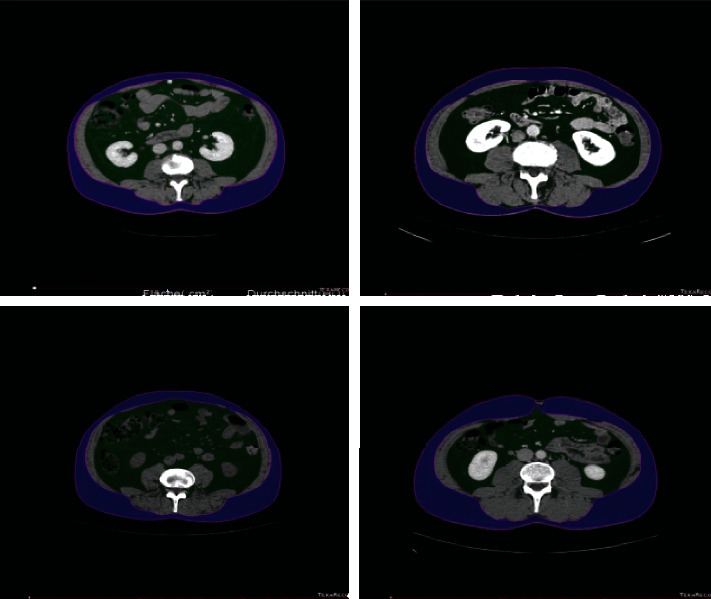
Manual segmentation of human fat CT images.
